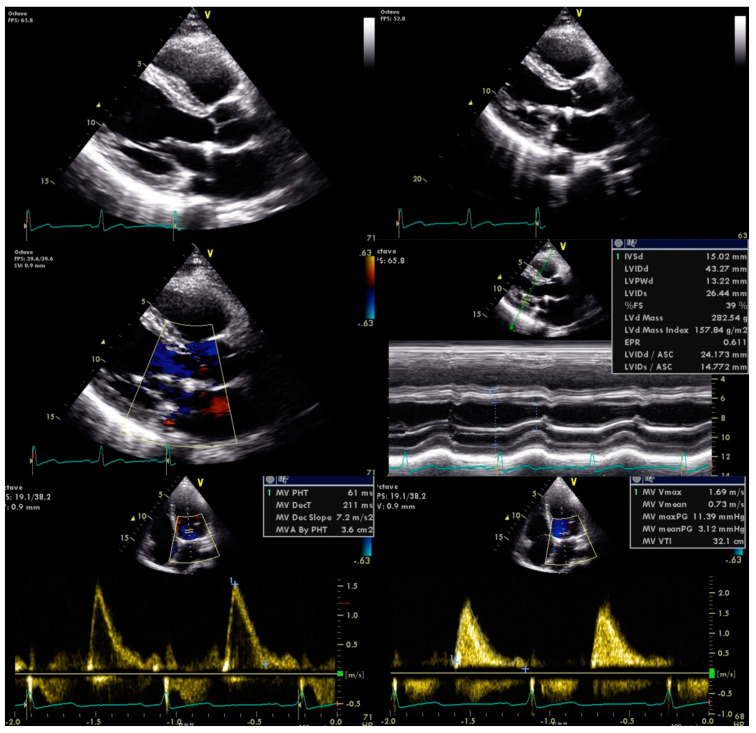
Transthoracic echocardiography post mitral valve plasty.
